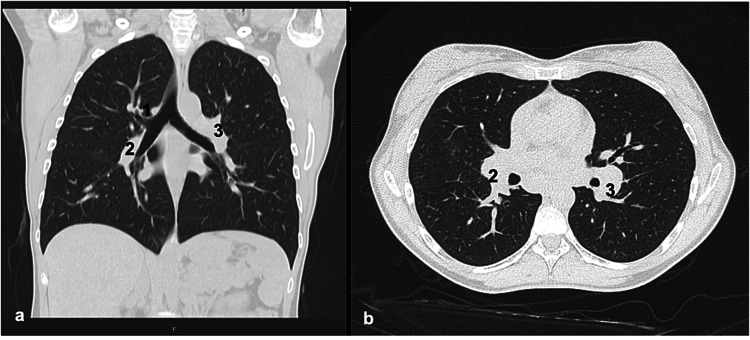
Contrast thoracic CT, in coronal (a) and transverse (b) plane, revealing a classic 123 sign with bilateral hilar (2 and 3) and paratracheal (1) lymphadenopathy, with no other abnormalities. CT, computed tomography
